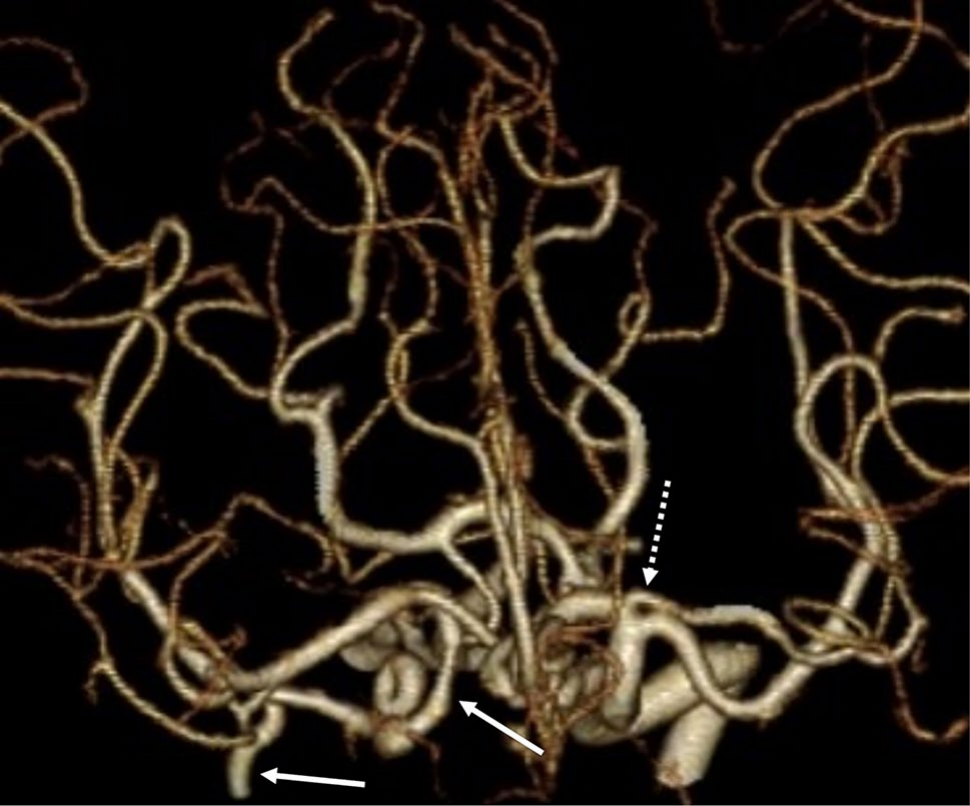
Anterosuperior to posteroinferior projection of CTA of intracranial region shows a left accessory MCA (*dotted arrow*) and a hypoplastic right ICA (*arrows*). Hyperplastic right posterior communicating artery and the right MCA is normal in size
